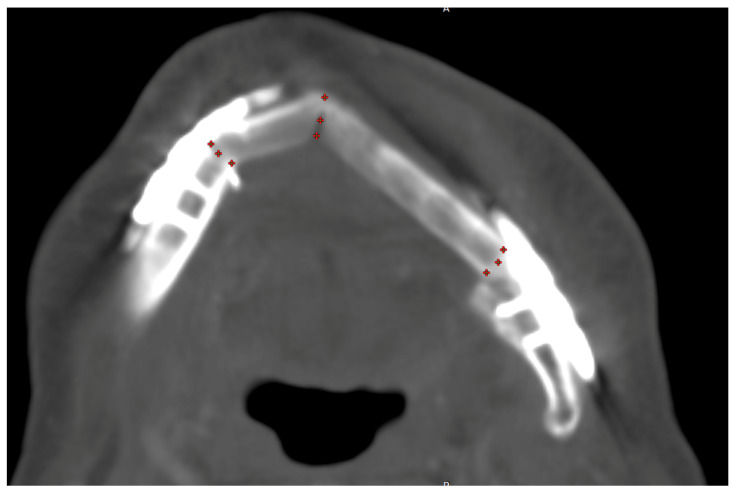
Measurement of Hounsfield Units in the CT scans: measurement of HU in the gap of all contact points with three ROIs each.
